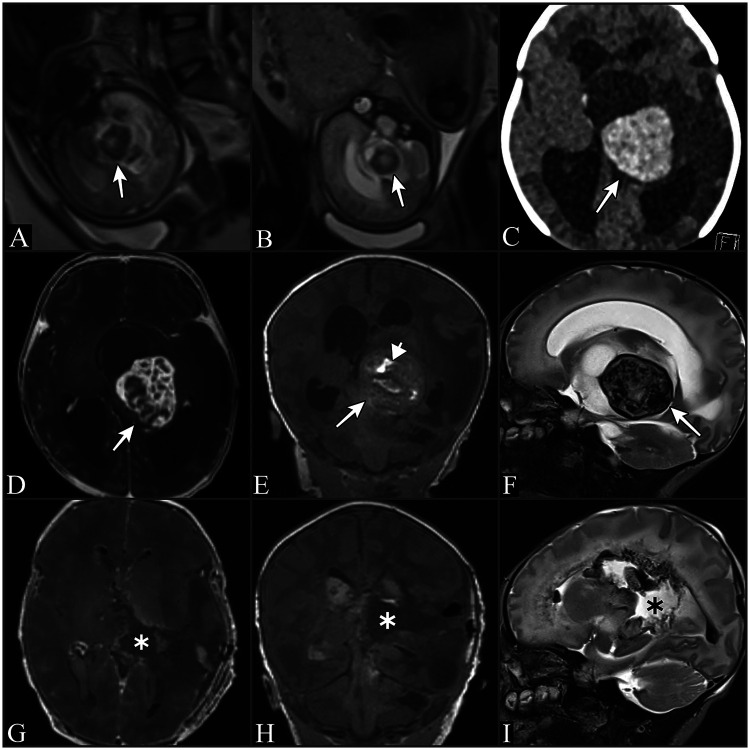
(A,B) fetal MRI of baby showing T2 hypointense mass lesion located in the midline structures of the brain (arrows). (C) Axial section cranial CT scan showing hyperdense mass lesion within left thalamus (arrow). (D) Axial section T1 weighted cranial MRI scan with contrast shows mass lesion with excess contrast uptake within left thalamus (arrow). (E) Coronal section T1 weighted MRI without contrast shows lesion (arrow) with hyperintense areas highlighting blood accumulation within the lesion (arrowhead). (F) The lesion was hypointense in T2 weighted images. (G) Axial section T1 weighted contrasted cranial MRI, (H) Coronal section T1 weighted cranial MRI without contrast, (I) Sagittal section T2 weighted image. (G,H,I) images shows gross total resection of the tumor (asterisks).
